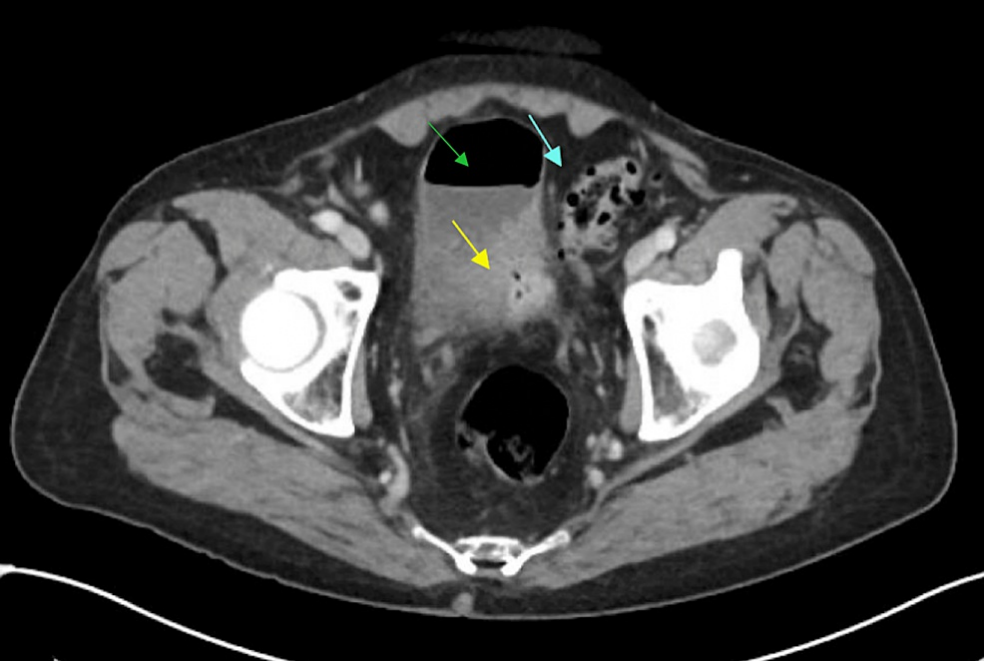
Axial computed tomography of the abdomen and pelvis with intravenous and oral contrast (Gastrografin). Gas locules (yellow arrow) and anti-dependent gas (green arrow) within the bladder and adjacent sigmoid diverticulitis (blue arrow) consistent with colovesical fistula.
